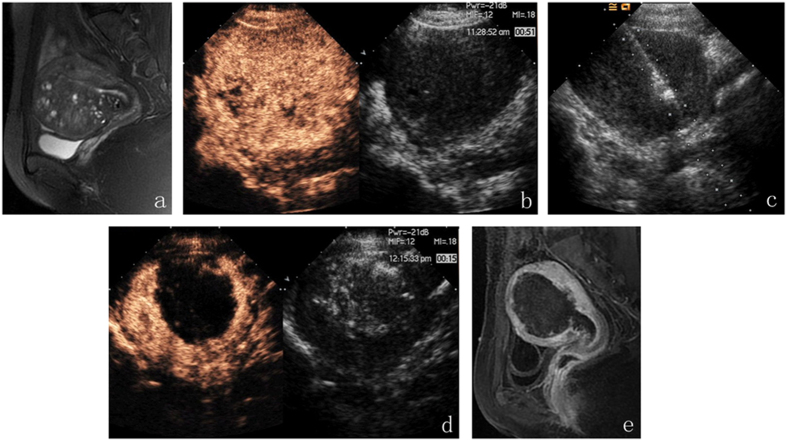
A 35-year-old woman with adenomyosis. Her uterus size was 9.3 cm*8.0 cm*8.7 cm. (a) MRI (T2WI): the enlargement of the junctional zone in the anterior wall. (b) CEUS before ablation: contrast agent perfused in the entire uterus. (c) The microwave energy radiated from the emission tip. (d) After the hyperechogenic signal covered at least 50% of the entire lesion, CEUS was performed to assess the volume of the non-perfused region. (e) Three days after PMWA, contrast-enhanced MRI was performed to evaluate both the efficacy of the ablation and the viability of the organs surrounding the uterus.
